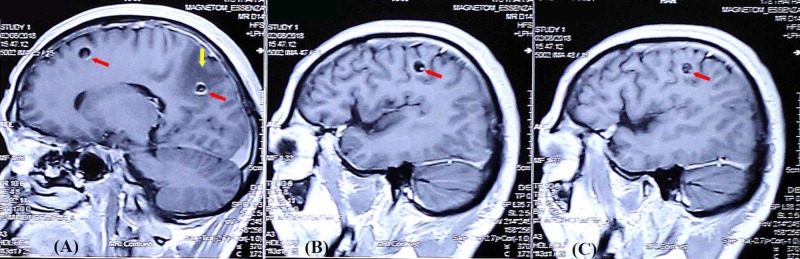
MRI findings of case 3 The sagittal T1 weighted image after contrast showed well-defined ring-enhancing cystic lesions (5-10 mm) in the right frontal lobe and parietal lobe with the scolex of *Taenia solium *cysticercosis inside (red arrows). The cystic lesion in the right temporal lobe had perilesional edema (yellow arrow) (A). A similar lesion located in the left parietal lobe was enhanced after contrast with a scolex of *Taenia solium* cysticercosis inside (red arrow) (B and C) MRI: magnetic resonance imaging
